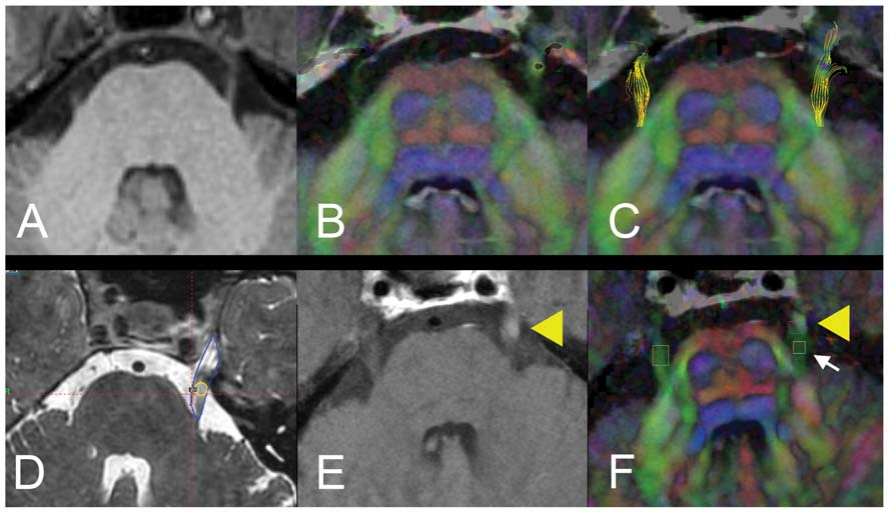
Baseline MR imaging, tractography of the trigeminal nerve, target and ROI definition. Image processing commenced with baseline anatomical 3TMR images (A, axial section, midpontine level). Diffusion tensor images with overlaid colour-by-orientation fibers are shown in B. Reconstructed tracts of the trigeminal nerve onto colour-by-orientation images are shown in C. Panel D depicts the contour of the trigeminal nerve (blue) and location of the radiosurgical shot. Yellow circle denotes the 80% isodose line, representing the “target” of Gamma radiation to the nerve. Panel E shows focal area of post-gadolinium enhancement on the trigeminal nerve (yellow arrowhead), defining the “target” ROI. Panel F shows the location of the “proximal” ROI, proximal to the area of gadolinium enhancement (B, white arrow), and “unaffected” ROI, contralateral nerve.
